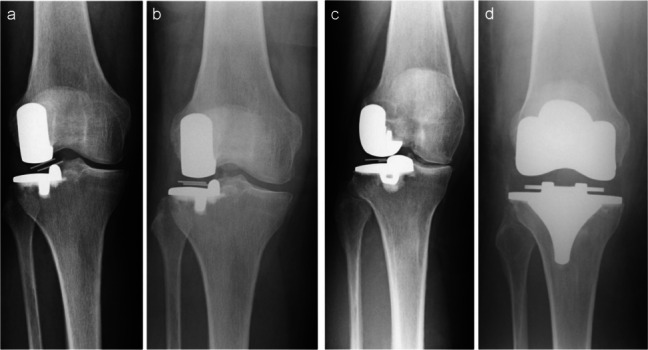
a A. p. radiograph demonstrating a bearing dislocation after mobile-bearing lateral UKA (b) Postoperative a. p. radiograph after revision surgery with bearing exchange (c) A. p. radiograph demonstrating progression of OA in medial compartment after mobile-bearing lateral UKA (d) Postoperative a. p. radiograph after conversion to TKA
